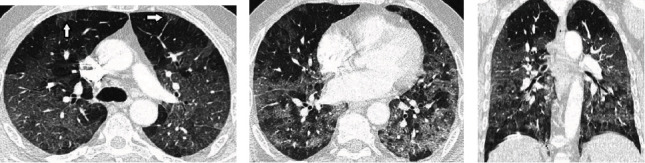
Radiological evaluation of severe COVID-19 pneumonia in a patient with DI genotype. A 60-year-old man with severe headache admitted to the emergency and had a positive RT-PCR test result for SARS-CoV-2. Thin section thorax CT images ((a, b) axial images, (c) coronal reformat) show bilateral diffuse ground-glass opacities with superimposed interlobular septal thickening (arrows) representing severe COVID-19 pneumonia.
